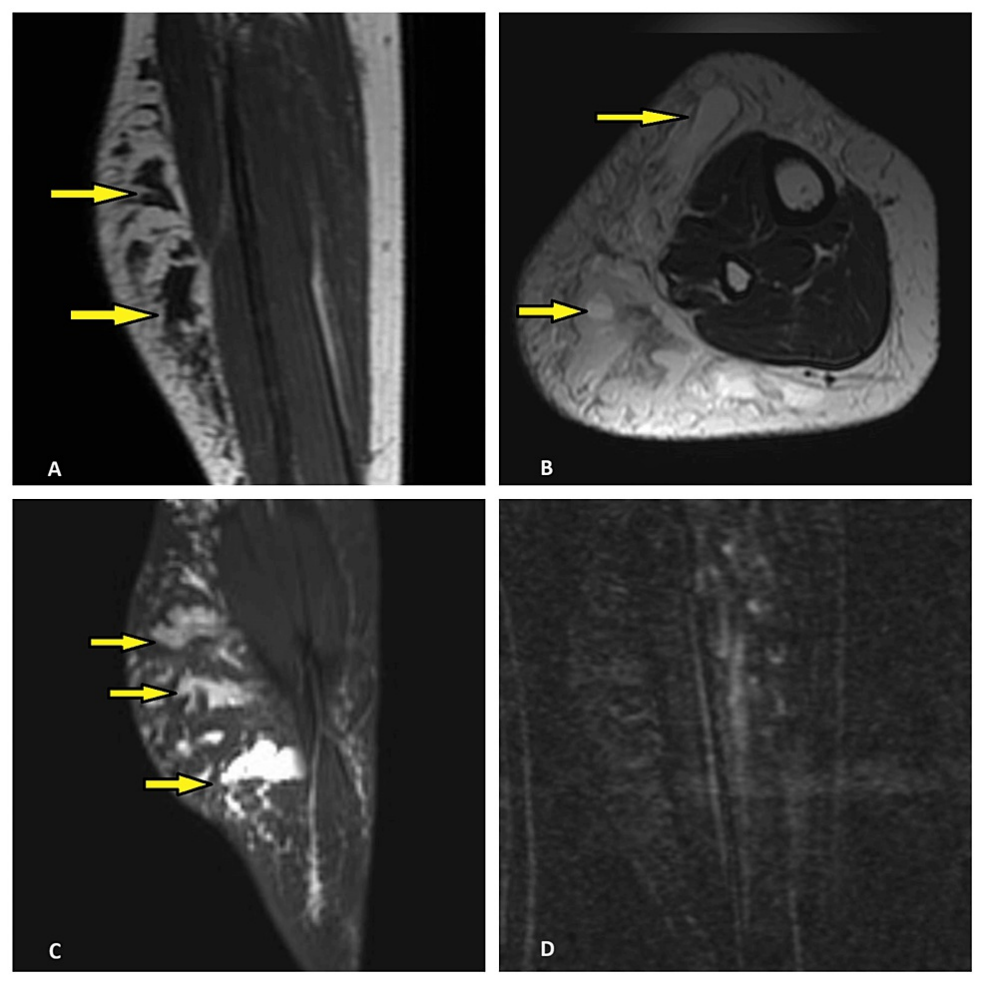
(A): Sagittal T1-weighted MRI image showing large, ill-defined multiloculated cystic lesion (indicated by yellow arrows) in the subcutaneous plane in the posterior aspect of right leg appearing hypointense on T1-weighted image. (B): Axial T2-weighted image showing multiloculated cystic lesion (indicated in yellow arrows) in the anterolateral and posterior aspect appearing hyperintense on T2-weighted image. (C): The lesion appears hyperintense in the sagittal STIR image (indicated by yellow arrows). (D): On post-contrast study, no enhancement is noted STIR: Short tau inversion recovery
